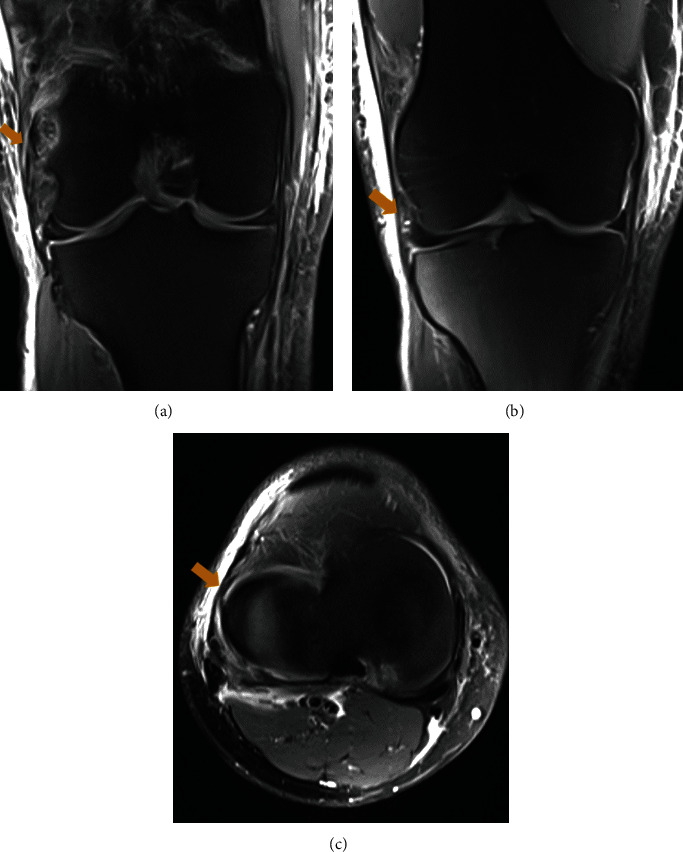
Patient case 2. (a, b, and c) Proton density coronal and axial MRI of the right knee at day 3 showing a loose though intact ALL (gold arrows).
